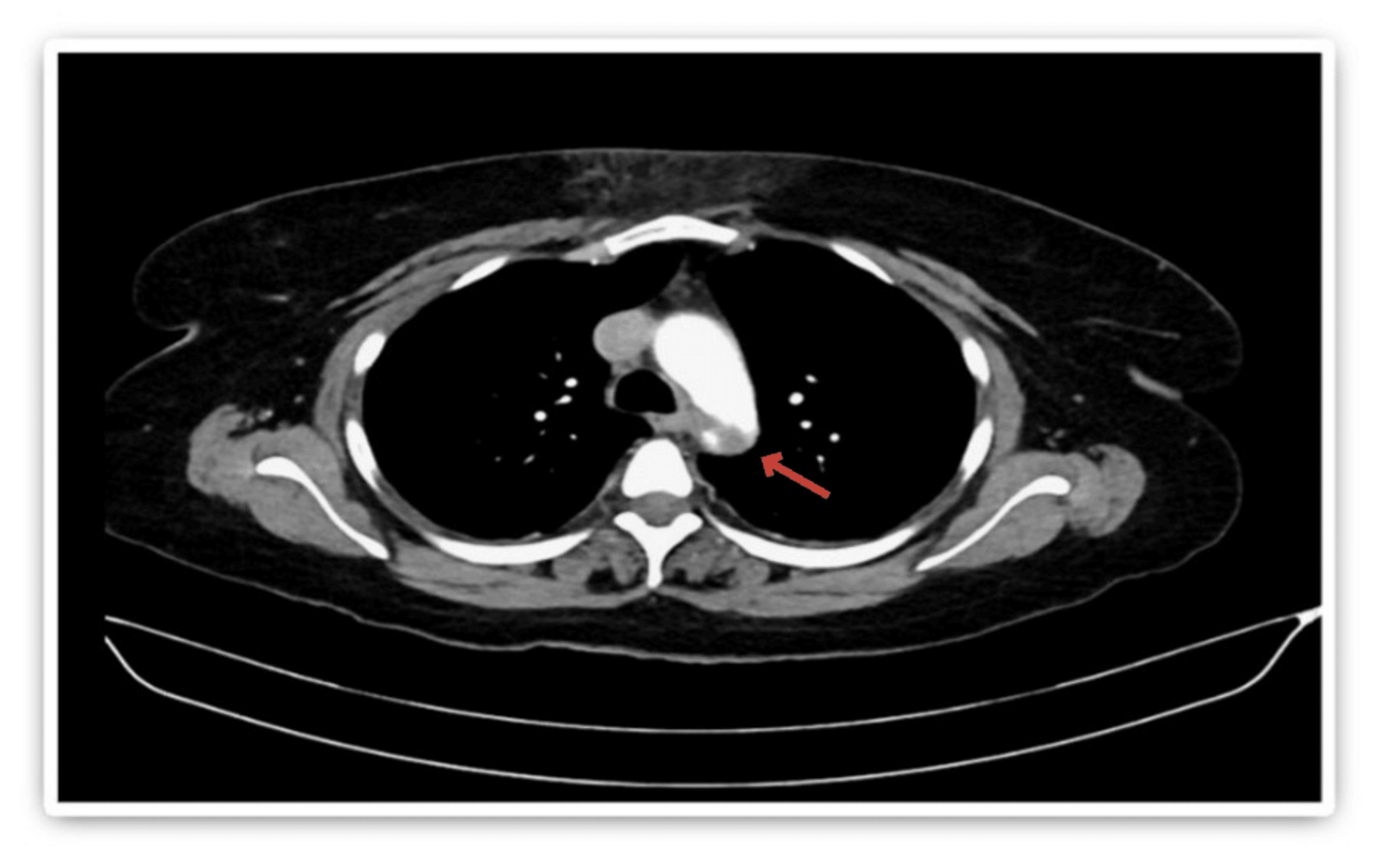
Axial section of CT chest showing an eccentric mural thrombus along the superior aspect of the distal thoracic aortic arch.
